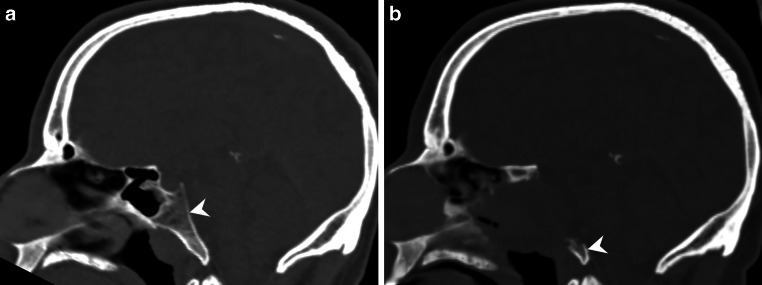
Sagittal reconstructions of CT images in bone window settings at initial presentation (a) and at the time of the last biopsy (b) show the osteodestructive features of the lesion with almost completely destroyed clivus (a + b, *arrowhead*)
